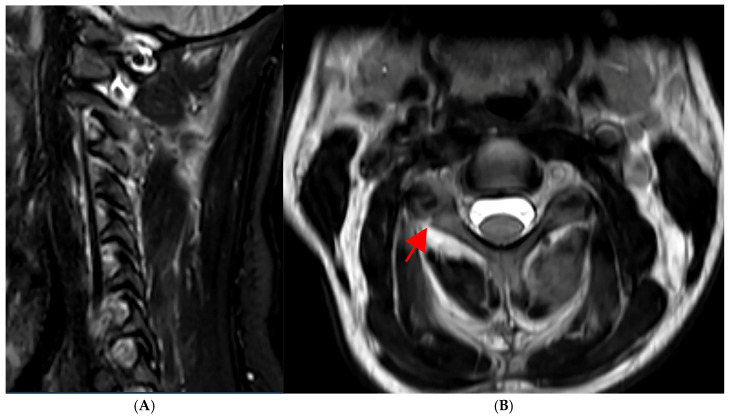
(A,B): MRI scan after emergency admission following a motor vehicle accident. (A) Sagittal FLAIR- and (B) axial T2-weighted scans documenting a diastatic fracture of the right facet and pedicle of C3 (AO Spine C3, F3, N0) without spinal compression (red arrow).
